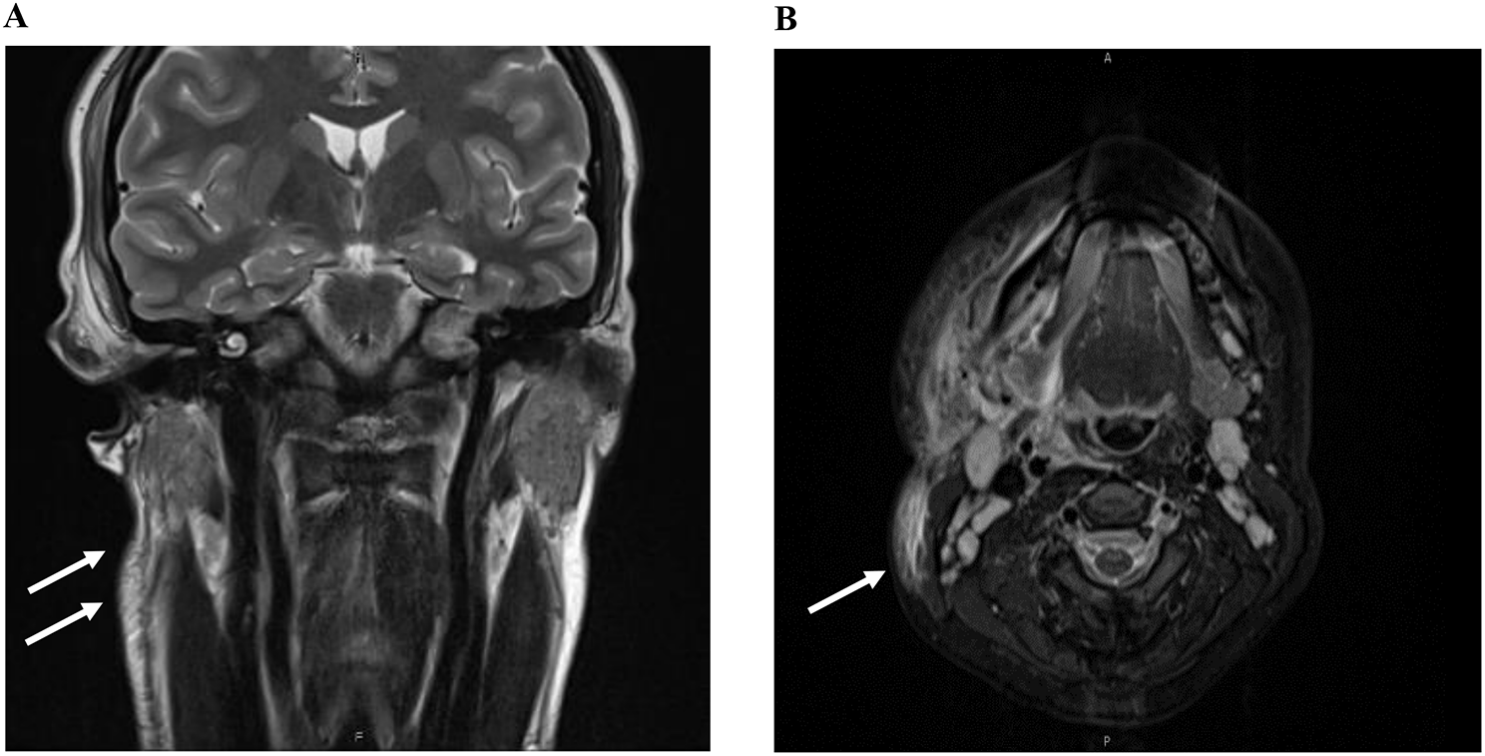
MRI sinuses without contrast showing white arrows pointing right sided myositis and parotitis in coronal (A) and right sided EJV thrombophlebitis in axial images (B)
